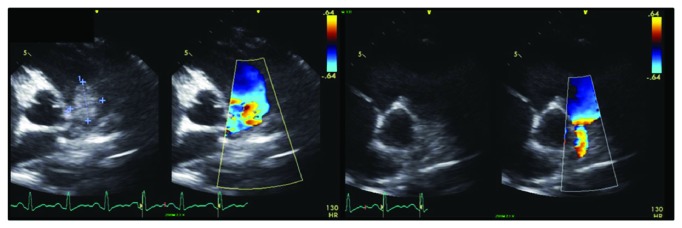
Transthoracic 2D echocardiography images. Echo images concerning for compression of the pulmonary vasculature.
